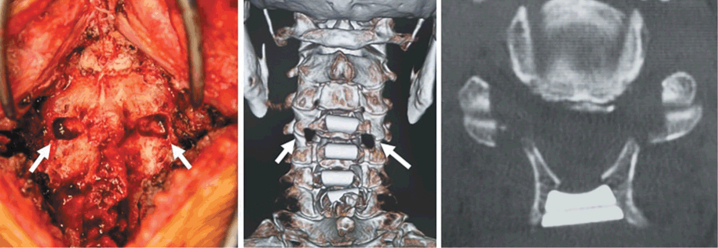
Cervical laminoplasty with prophylactic bilateral C4/5 foraminotomy. Left: Photograph showing the foraminotomy (arrows). Middle: Anteroposterior three-dimensional computed tomographic image showing the foraminotomy (arrows). Right: Axial computed tomography.
